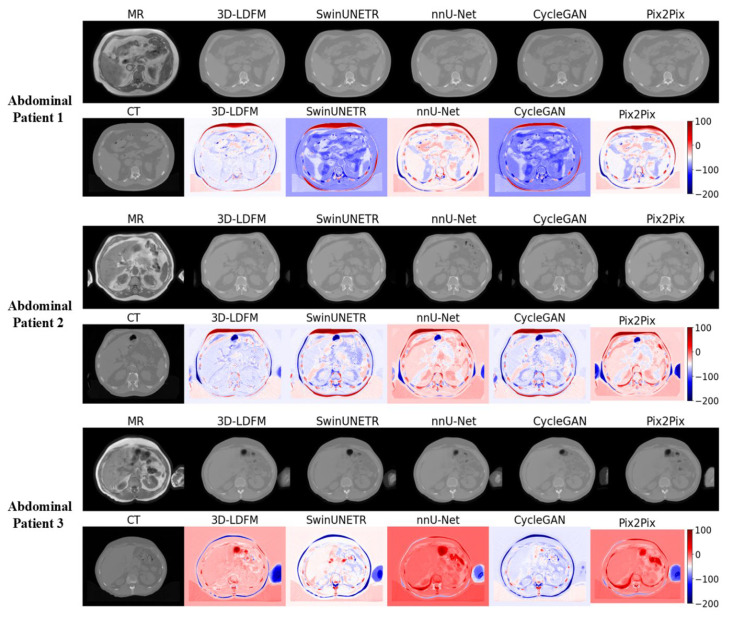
Abdomen (Pelvis/Lower Abdomen Region). Qualitative comparison and error maps for sCT generation in the pelvis/lower abdomen region. Rows show MR input, ground-truth CT, and sCT predictions from multiple methods, followed by HU-difference error maps. 3D-LDFM demonstrates the most accurate soft-tissue modelling, reflected in lower-magnitude and less saturated error maps.
